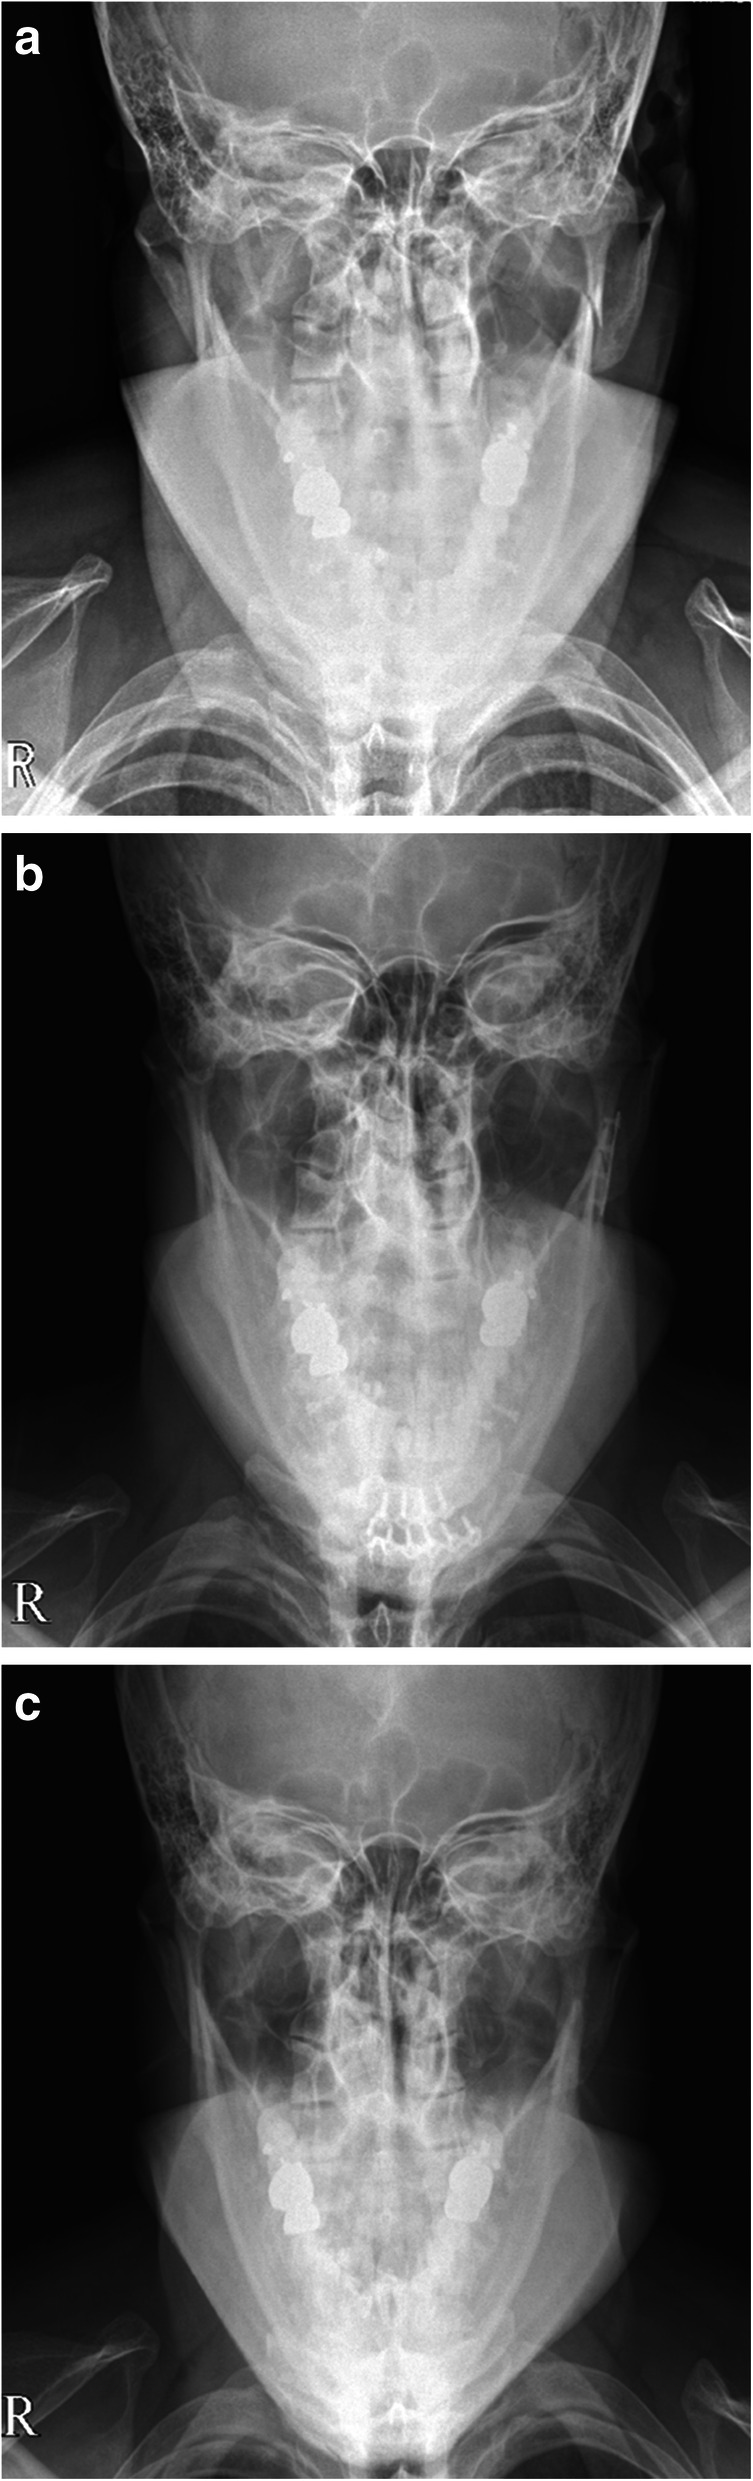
A 31 year-old male with a left subcondylar fracture of the mandible was treated using the modified intraoral approach. Modified Towne’s radiographic images show a laterally deviated subcondylar fracture (a) and the postoperative reduction state of the fracture following rigid internal fixation (b). Eight months after surgery, the plate and screws were removed through an incision made at the previous oral incision scar, without an external incision (c)
